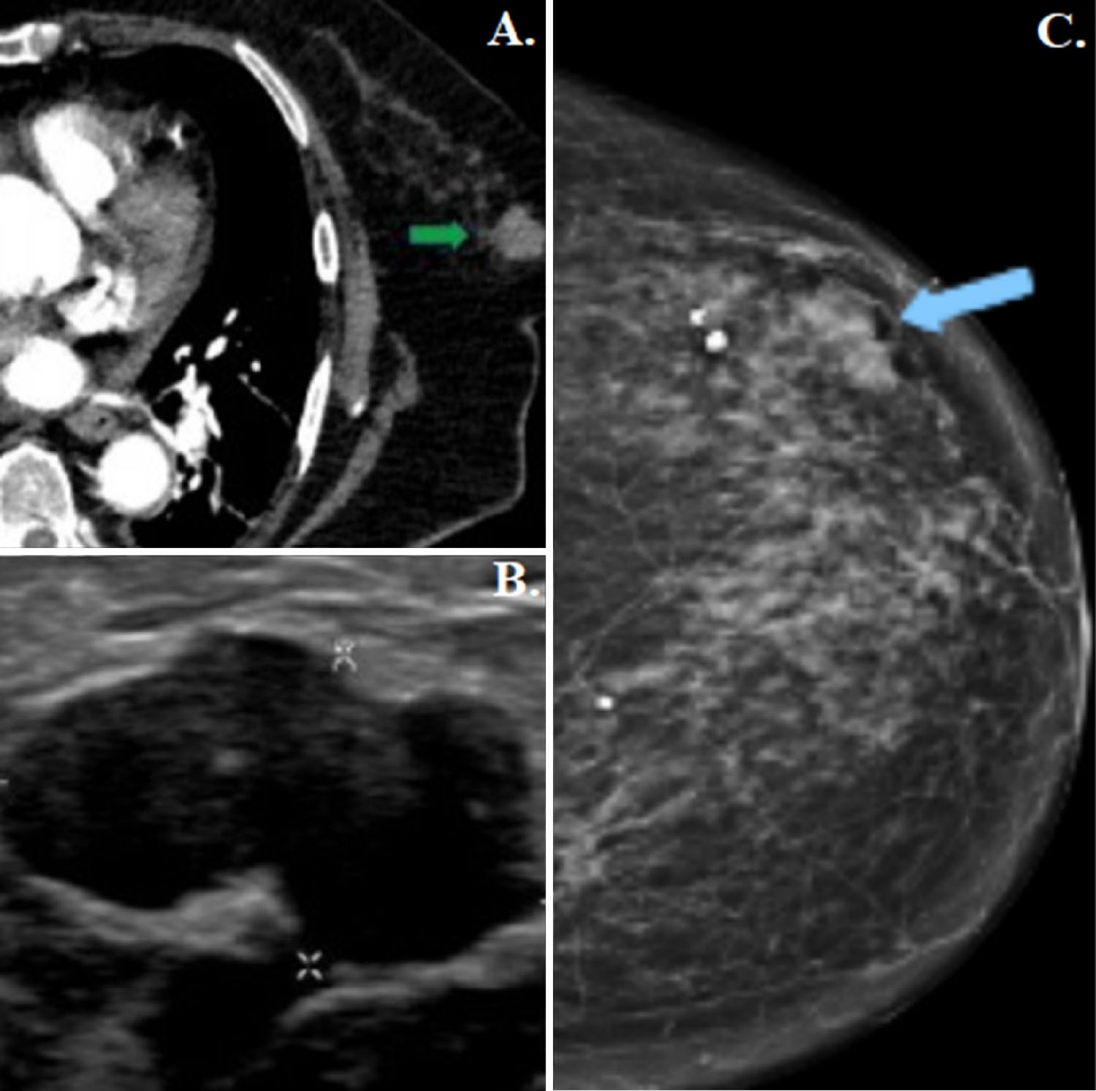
Fibroadenoma. A Left breast tissue CT imaging after organic iodinated contrasted agent intravenous injection presenting a nodular tumour with well-defined and lobulated margins lacking marked contrast enhancement (green arrow). B Mammary ultrasound showing a hypoechoic tumour with sharp and polylobed margins. C Mammogram in cranio-caudal projection showing a homogenous mammary thickening at the junction of the left breast outer quadrants (blue arrow)
